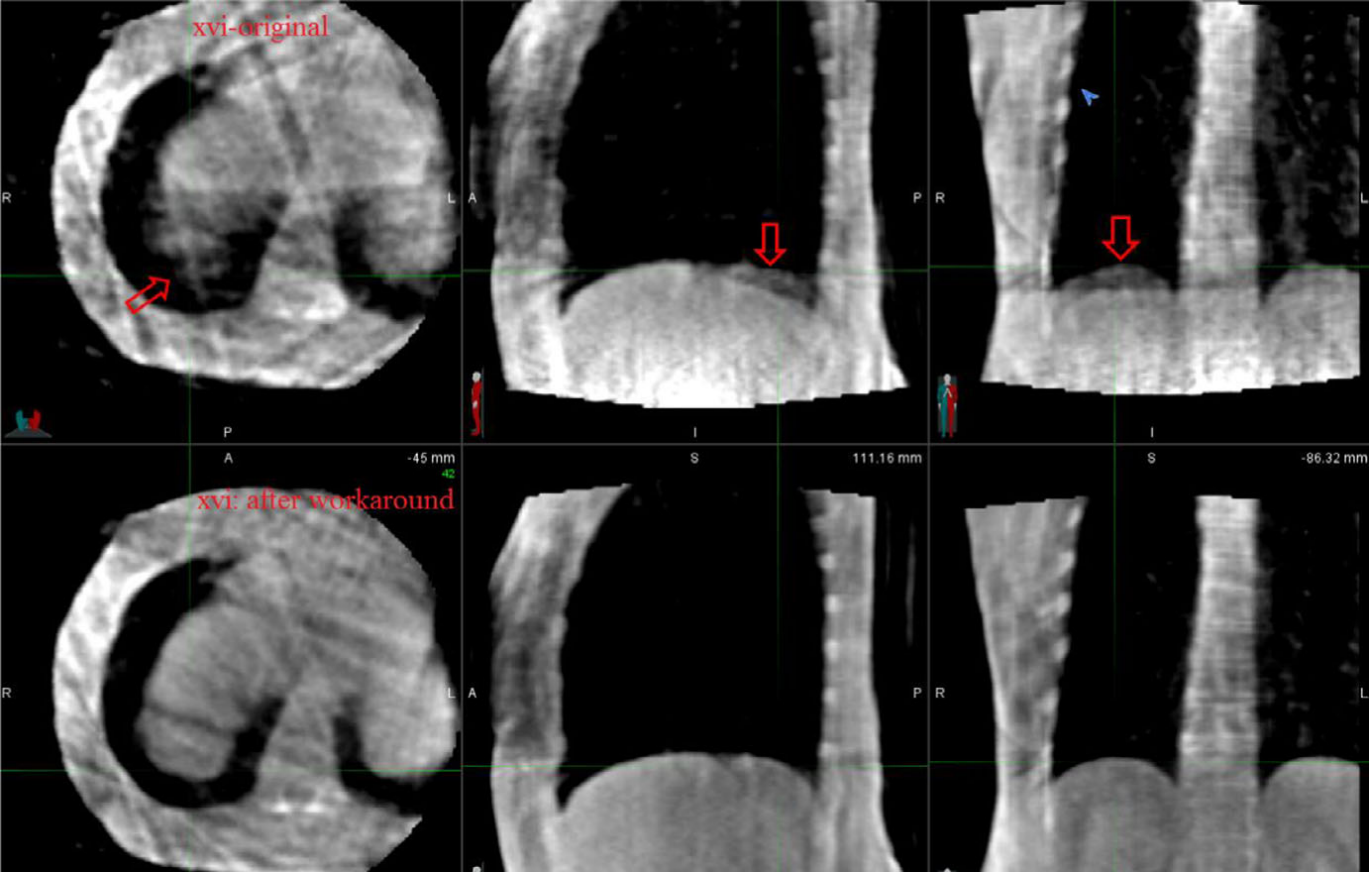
Comparison of intrafraction four‐dimensional cone beam computed tomography images for a stereotactic body radiotherapy lung patient with and without XVI file modification workaround. Same Window and Level was applied for both image displays. Without workaround there is significant blurring of the diaphragm position highlighted by the red arrows making tracking of the diaphragm position difficult.
